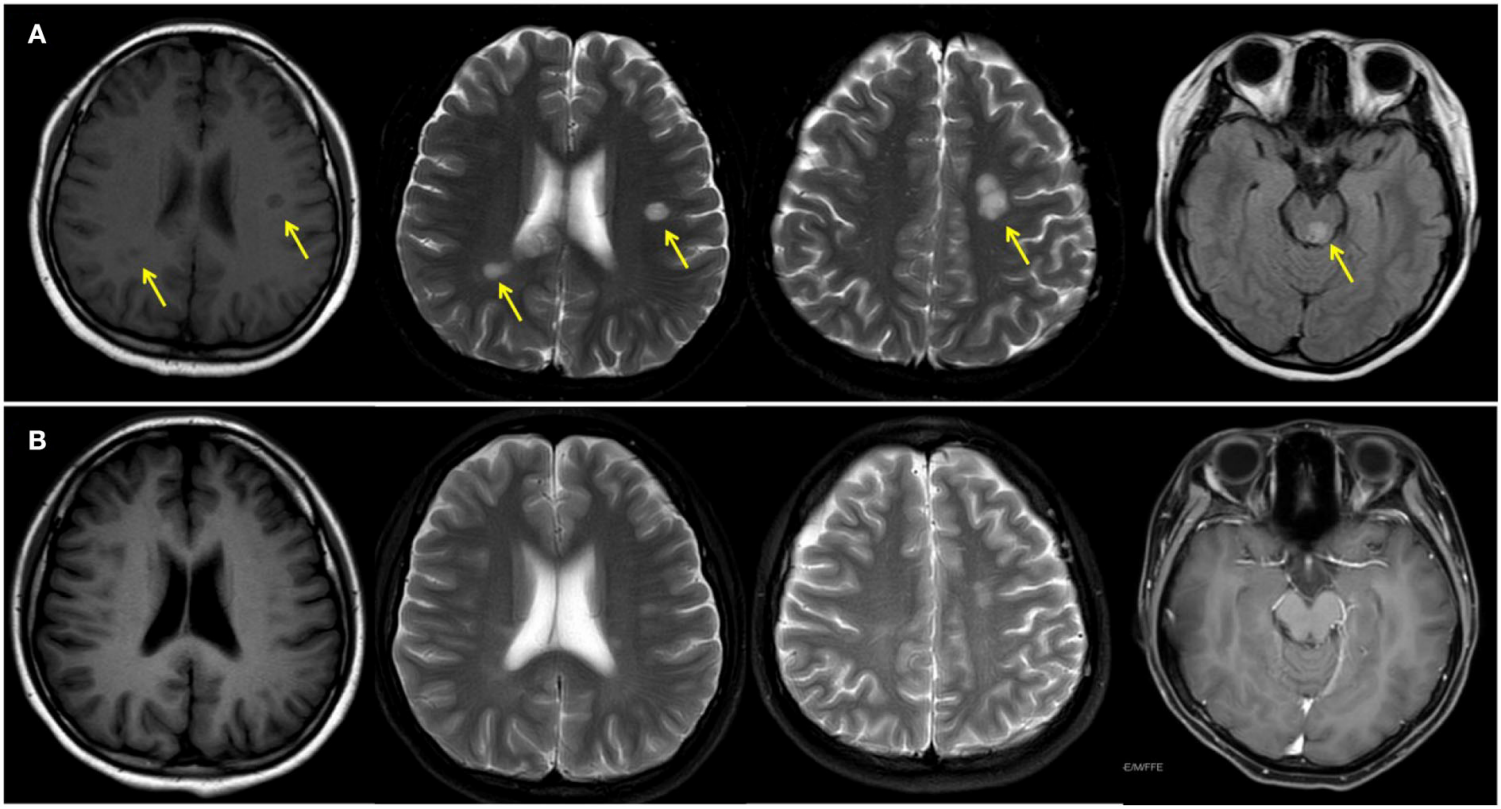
Magnetic resonance imaging of the brain before (A) and after (B) treatment. Yellow arrows indicate white matter lesions.
